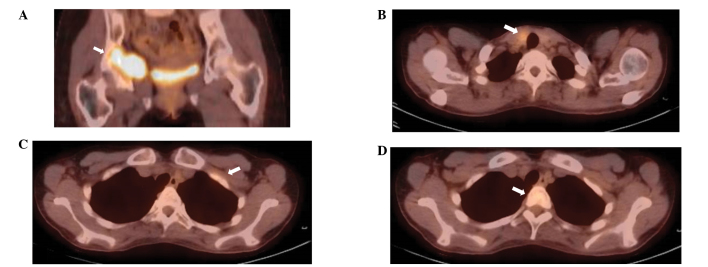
Case one: Positron emission tomography with ^18^F-fluorodeoxyglucose (FDG-PET) showing abnormal uptake in the (A) right acetabulum, (B) right lobe of the thyroid gland, (C) left first rib and (D) T2 vertebral body, which were considered to be multiple bone metastases from the thyroid cancer.
